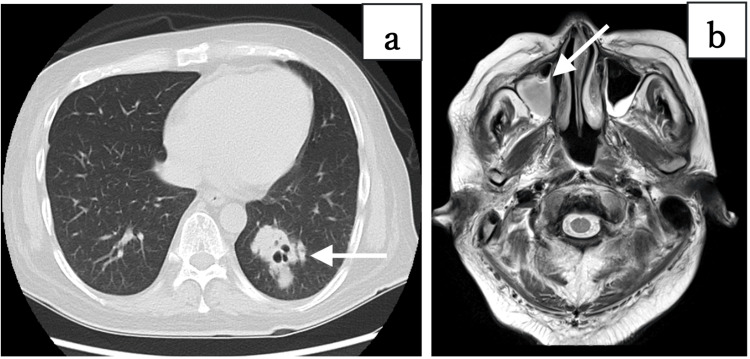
(a) A well-defined cavitary nodule was observed in the left middle lobe, as indicated by the white arrow on the chest CT. (b) The head MRI scan revealed fluid accumulation in the right maxillary sinus, as indicated by the white arrow on the T2-weighted image CT: computed tomography; MRI: magnetic resonance imaging
